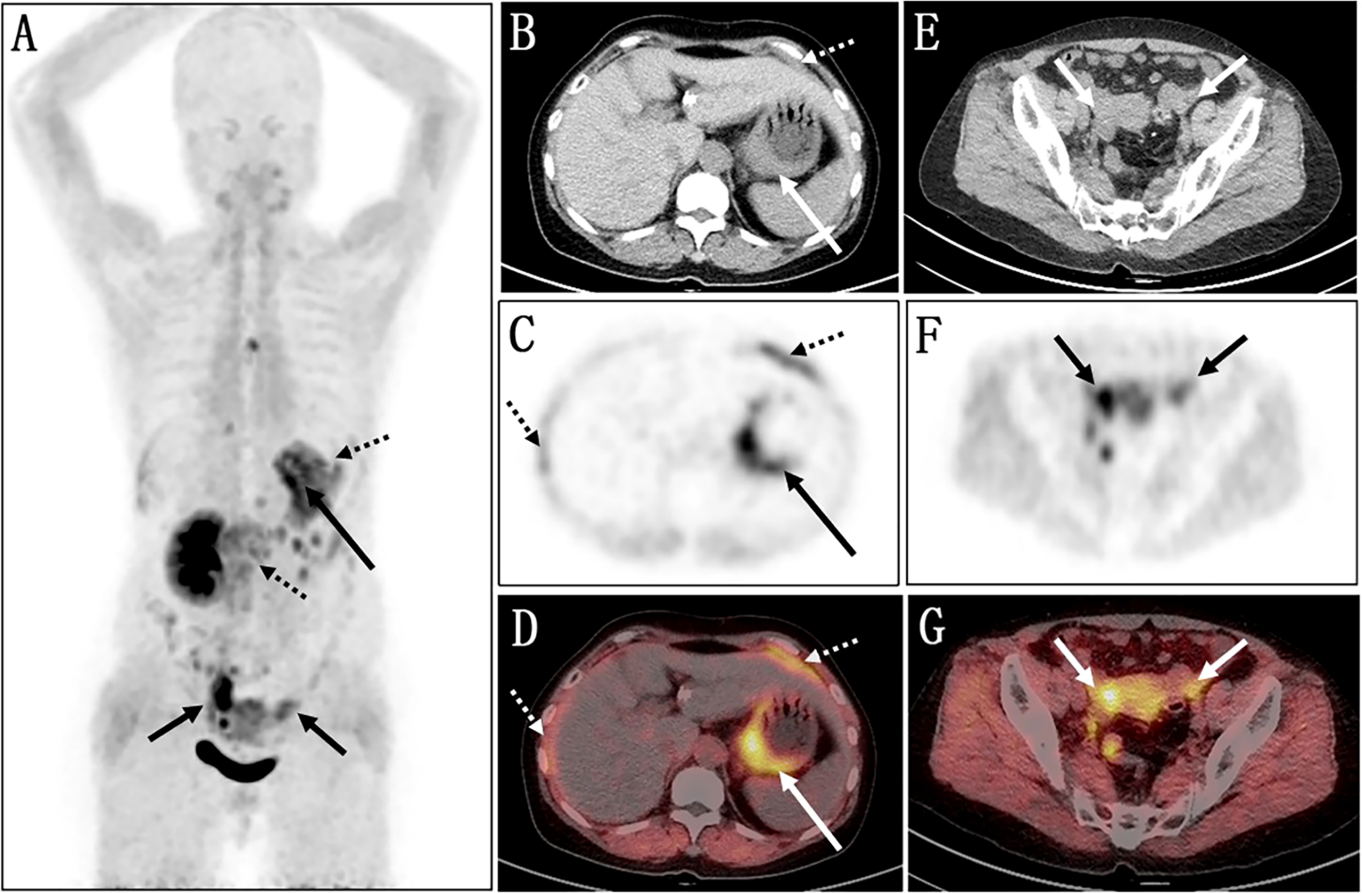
Images of ^68^Ga-FAPI PET/CT. (A) The MIP image shows several lesions with varying degrees of FAPI uptake. (B–D) Axial views of abdominal cavity demonstrate significantly elevated FAPI activity in the thickened gastric wall (long arrows; SUV_max_ 9.8). (E–G) The axial images of pelvic cavity reveal abnormal FAPI uptake in the bilateral adnexal areas (short arrows; SUV_max_ of right 11.6, SUV_max_ of left 6.5). (C, D) Furthermore, ^68^Ga-FAPI PET/CT showed extensive peritoneal carcinomatosis with FAPI uptake (dotted arrows; SUV_max_ 3.9).
